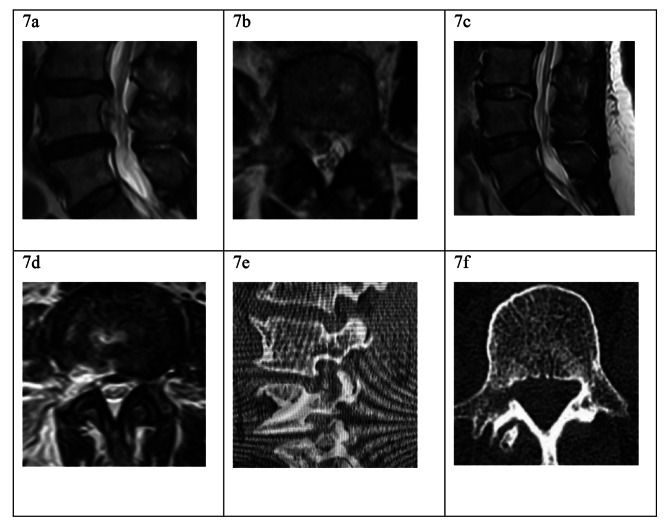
A case presentation for very-highly downward-migrated herniation. (a): The preoperative sagittal MRI showing a very-highly down-migrated fragment along the posterior L4 vertebra; (b): The preoperative axial MRI showing the migrated fragments hidden in the L4 medial pedicle; (c), (d): The postoperative sagittal and axial MRI showing that the fragments were completely retrieved, the L4/5 disc was maintained intact; (e), (f): The postoperative sagittal and axial CT showing the partial pediculectomy, while there is no pedicle fracture
